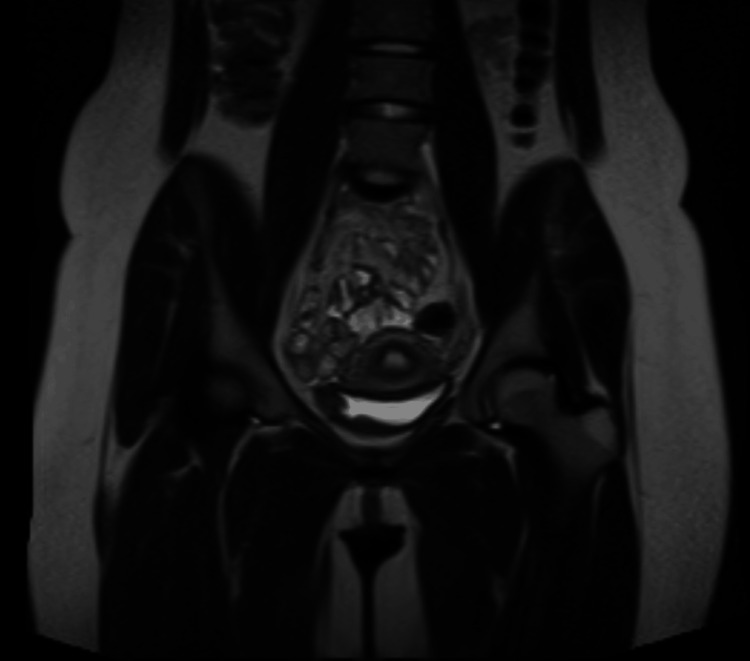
Post-operative contrast-enhanced pelvic MRI T1 coronal
